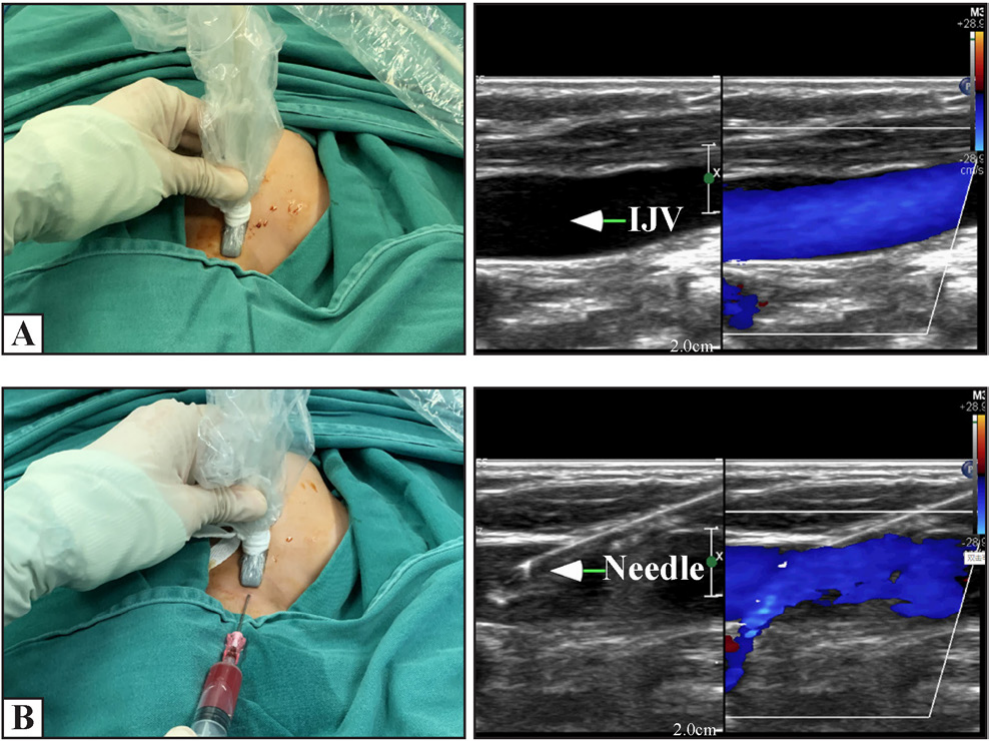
Long-axis in-plane ultrasound-guided IJV puncture. (A) The long axis of the IJV is displayed in the ultrasound image. (B) The needle passes through the anterior wall of the vessel, and the blood can be aspirated. IJV, internal jugular vein.
